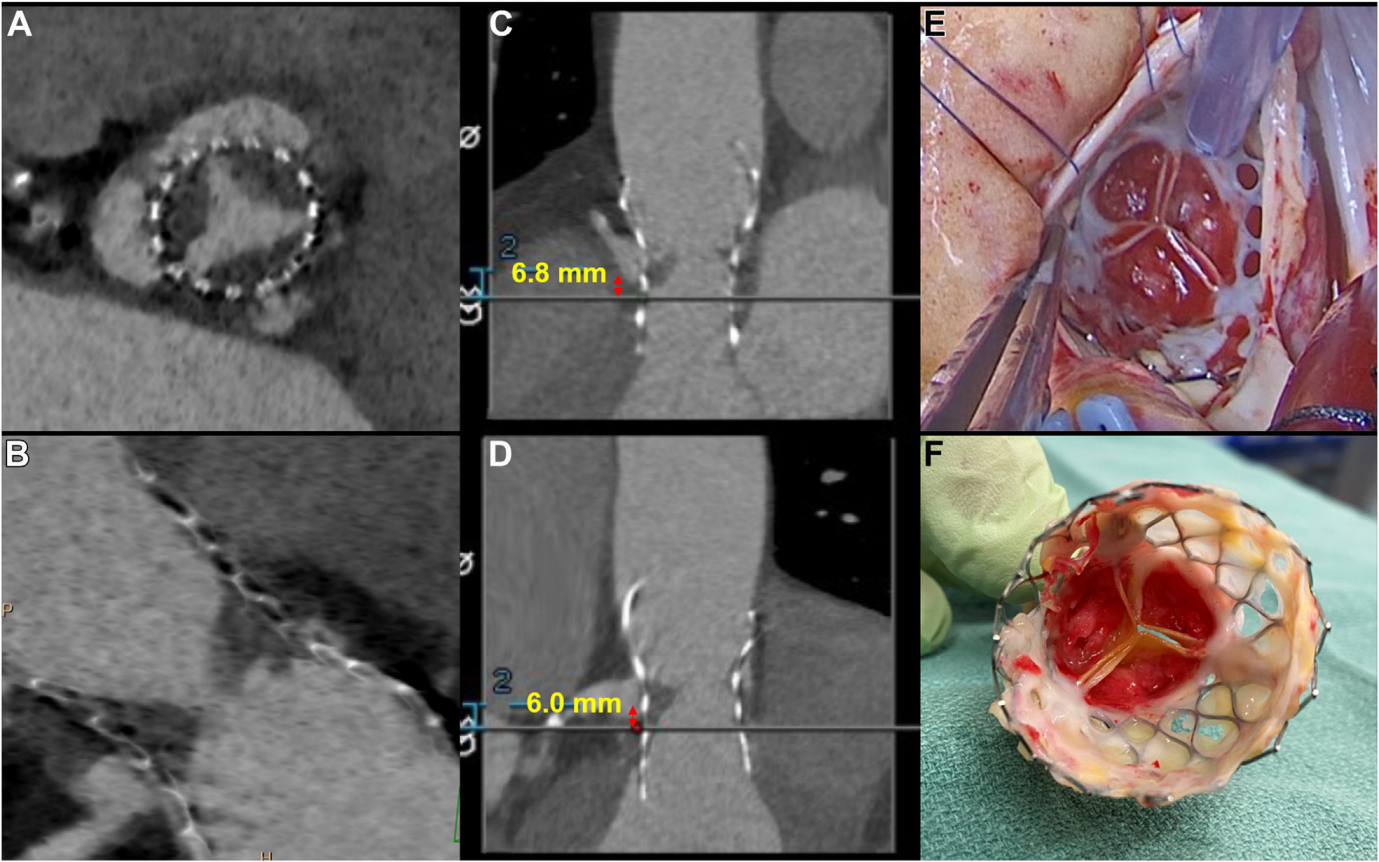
Transcatheter Aortic Valve Thrombosis (A) Short-axial and (B) long-axial computed tomography demonstrated 3-leaflet thrombosis of the transcatheter aortic valve and low coronary heights of the (C) right coronary artery and (D) left coronary artery. Intraoperative inspection of the transcatheter aortic valve showed thrombosis of all leaflets on both the inflow and the outflow (E and F).
